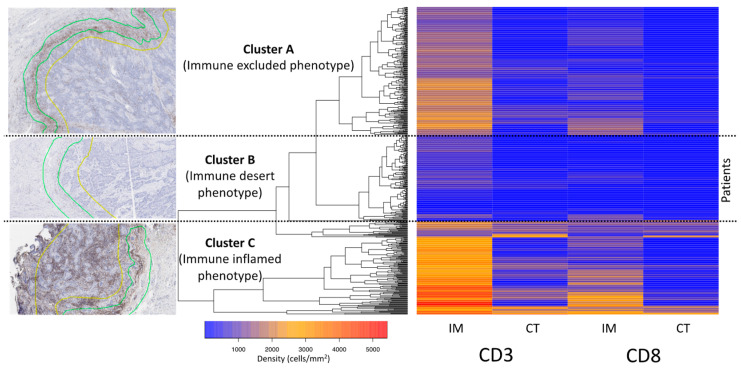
Unsupervised hierarchical cluster analysis of vulvar cancer samples. The two-way (T-cell densities against samples) unsupervised hierarchical cluster of 530 vulvar cancer samples and the CD3^+^/CD8^+^ T-cell densities (rows) at the invasive margin (IM) and in the center of the tumor (CT) is shown. Representative images of the three major clusters are depicted next to the corresponding clusters.
